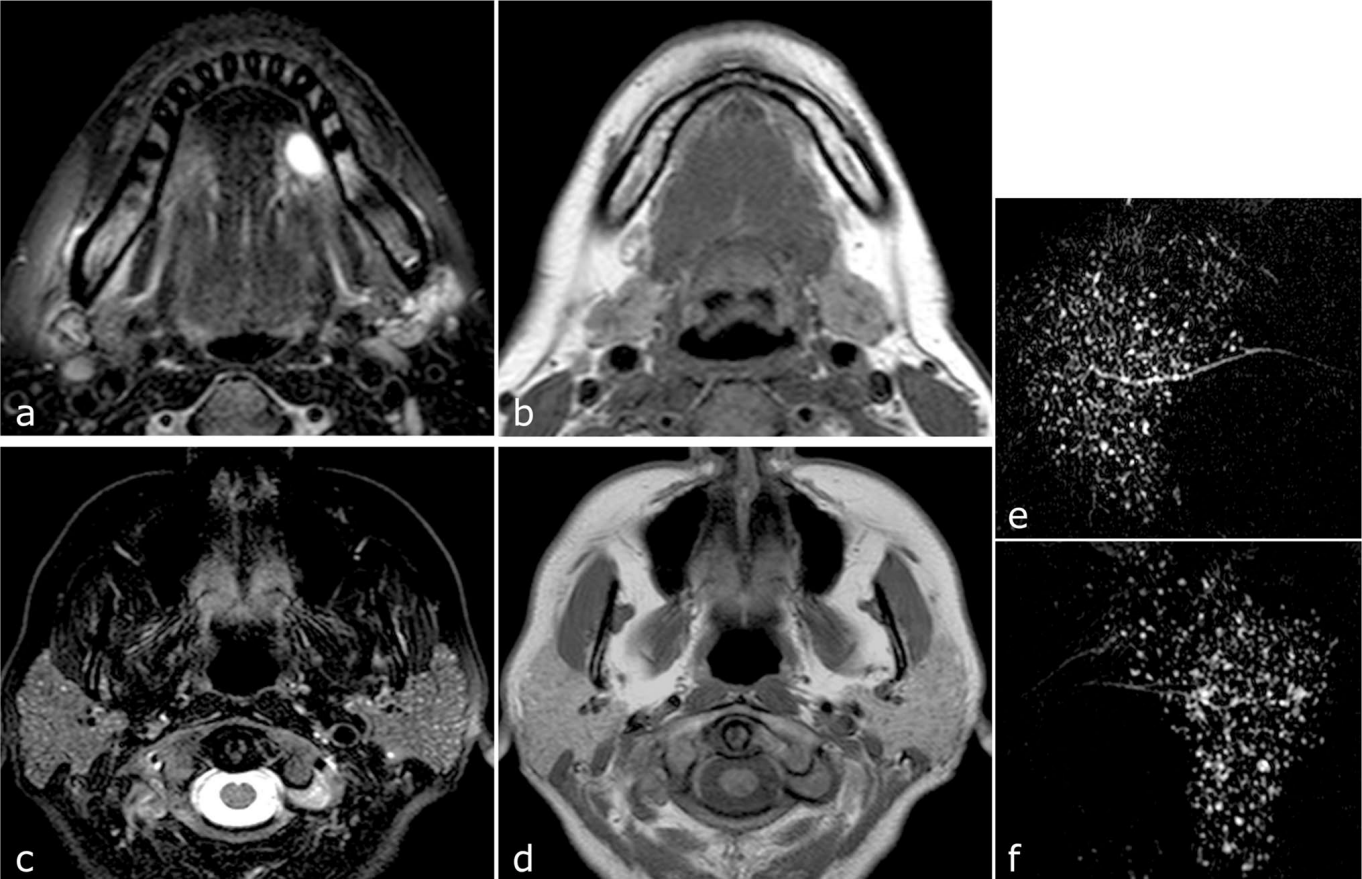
Magnetic resonance images of a 46 year-old woman with simple ranula who was diagnosed with pSS (Case 8 in Table 1). a Axial fat-suppressed T2-weighted MR images show a homogeneous, high-intensity, well-circumscribed cystic lesion in the left sublingual space. b Axial T1-weighted image shows relatively homogeneous parenchyma without fat degeneration of the bilateral submandibular glands. c Axial fat-suppressed T2-weighted image shows multiple very small high-intensity spots in parenchyma of the bilateral parotid glands. d Axial T1-weighted image shows relatively homogeneous parenchyma without fat degeneration of the bilateral parotid glands. e, f MR sialography shows punctate sialoectasia in the right (e) and left (f) parotid glands
